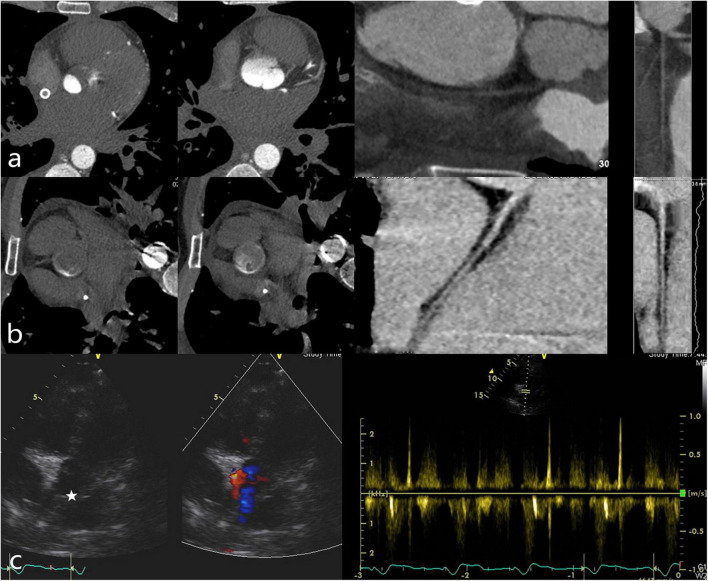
(a) Patient in the supine position. The axial view demonstrated an arc-shaped low-attenuation filling defect (“watershed” phenomenon) in the root of the aorta while the DAO was filling well. The multiplanar reformatted image from the CTA showed no contrast agent filling in the right coronary. (b) Patient in the right lateral decubitus position. The arc-shaped low-attenuation filling defect disappeared and the right coronary filled well. (Left dominant coronary artery; (c) The echocardiography (GE Vivid E95; 45° reclining position; Five-chamber view) demonstrated the extremely low velocity of the aortic valve and there was no thrombus formation in the aortic root (white star).
